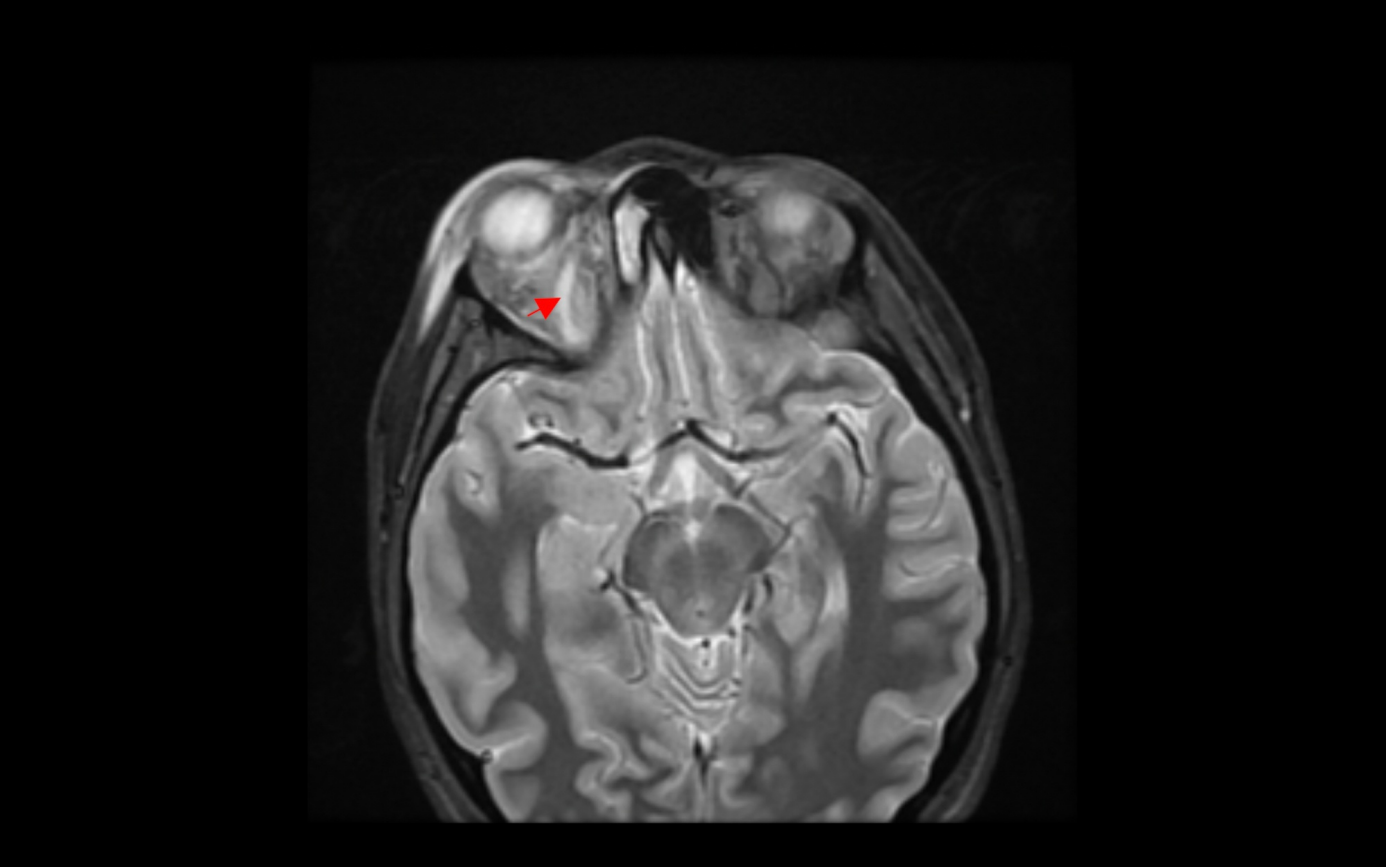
Axial STIR MRI without contrast showing thrombosis of the right superior ophthalmic vein with right orbital proptosis and enlargement of right lateral rectus. STIR, short tau inversion recovery
